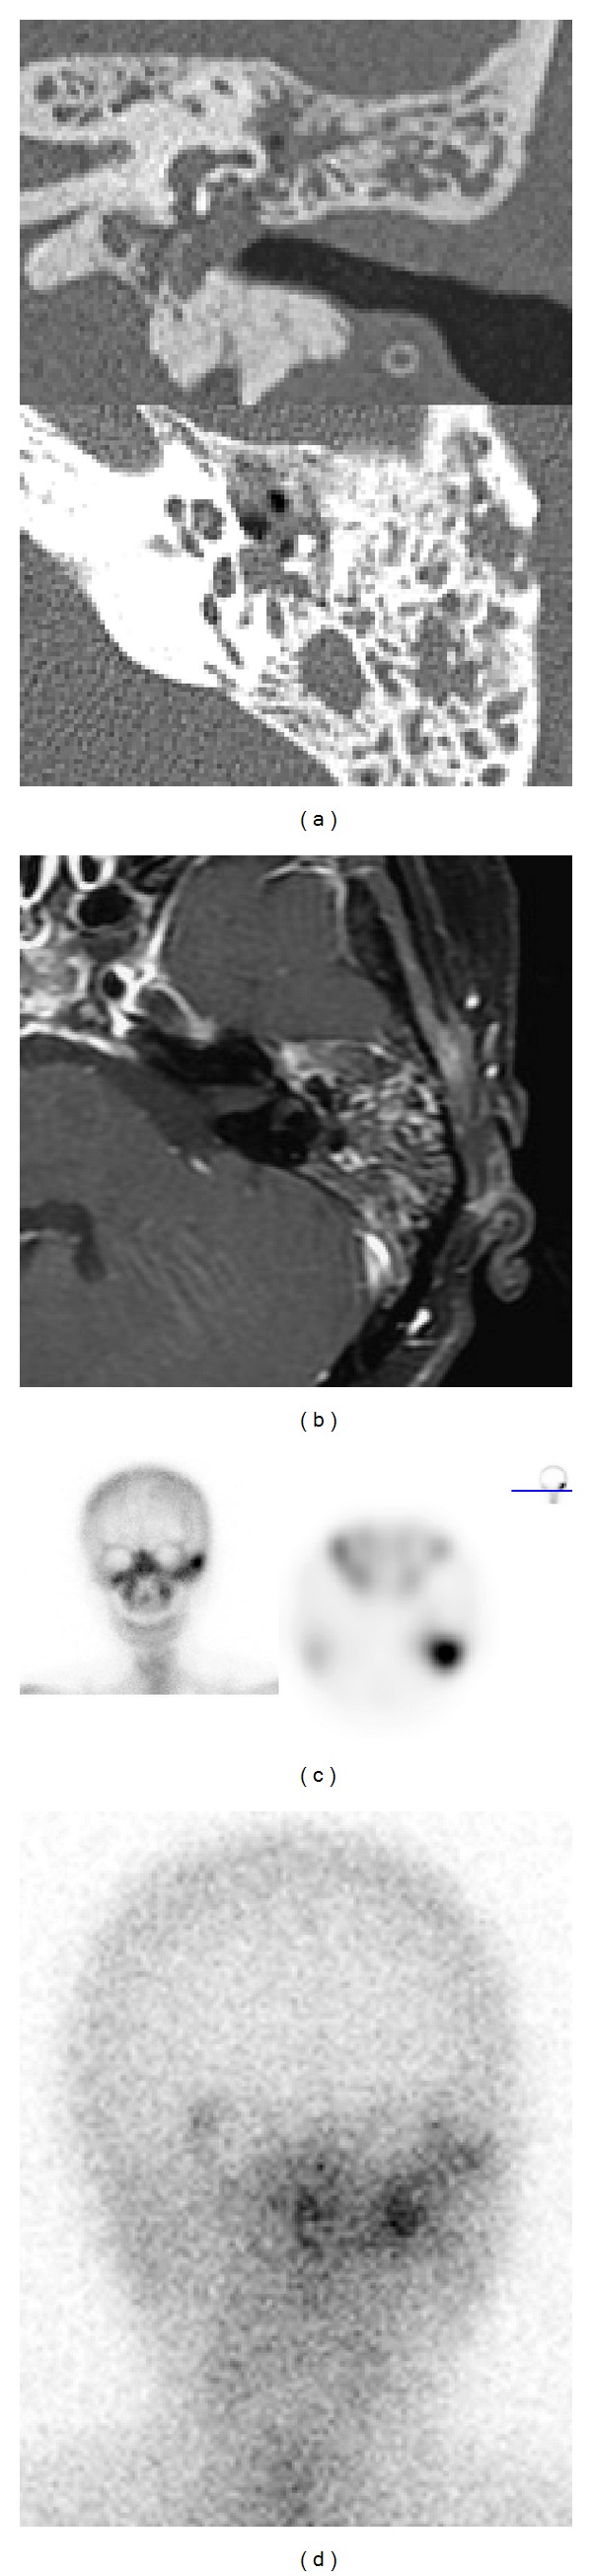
Images of a patient of temporal bone osteomyelitis after radiotherapy for nasopharyngeal carcinoma. (a) CT. (b) Contrast-enhanced T1-weighted MR. (c) Tc99m bone scan. (d) Gallium scan. See text for details.
